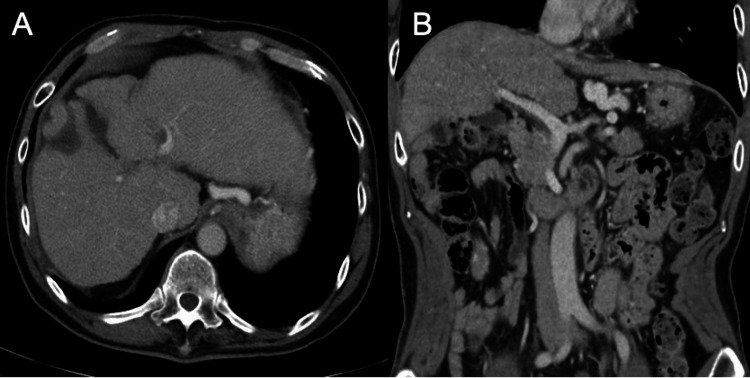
Findings of follow-up CT at three months postoperatively No recurrence of hepatocellular carcinoma was observed. The liver parenchyma shows stable appearance without evidence of new lesions or vascular complications.
